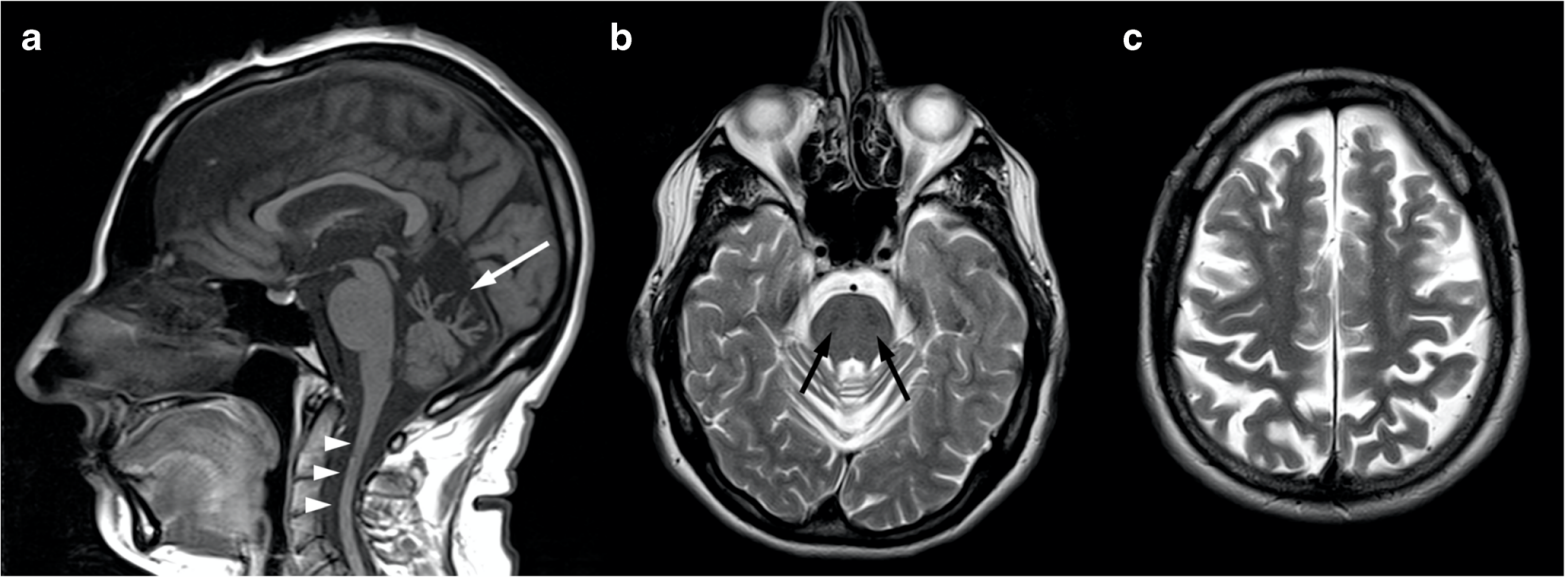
Brain MRI findings in a 45-year-old female ARSACS patient. A predominant superior vermian atrophy is shown on a sagittal T1-weighted sequence (a, white arrow), along with as a mild T2-weighted hypointensity affecting the transverse pontine fibers (b, black arrows). Parietal (c) and cervical spinal cord (a, arrowheads) atrophy is also evident
